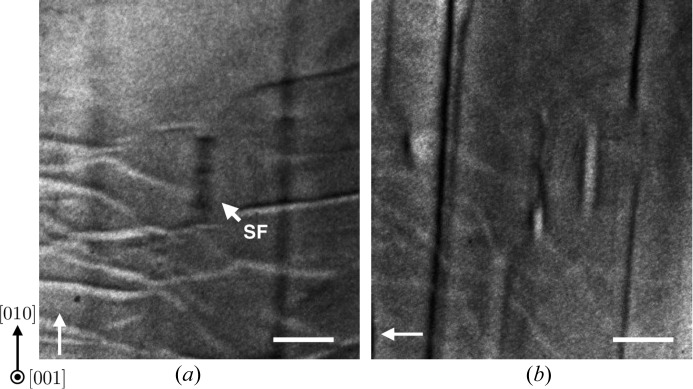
Comparison of X-ray topographs from a (001)-oriented wafer acquired with (*a*)
